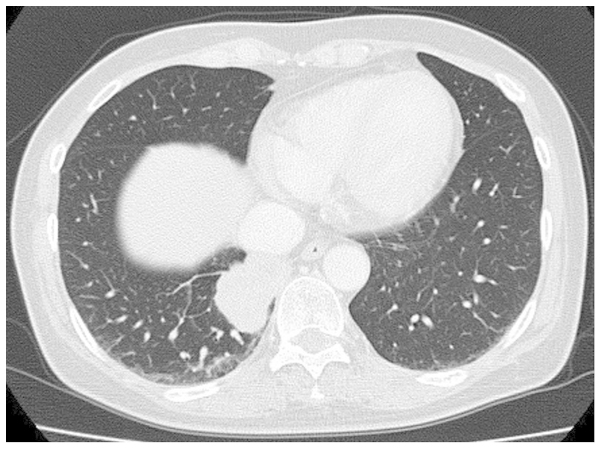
Chest computed tomography scan at the time of first admission showing a large mass in the right lower lobe of the lungs.
